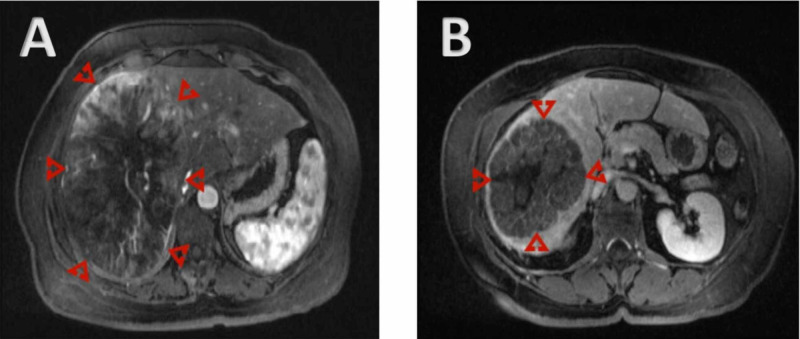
(A-B) MRI showing hypervascular liver mass with washout on delayed images A: Axial T1 FS C+ arterial phase; B: Axial T1 FS C+ delayed Case courtesy: Dr Ahmed Subaie, Radiopaedia.org, rID: 25953; https://radiopaedia.org/cases/hepatocellular-carcinoma-13?lang=us MRI, magnetic resonance imaging
